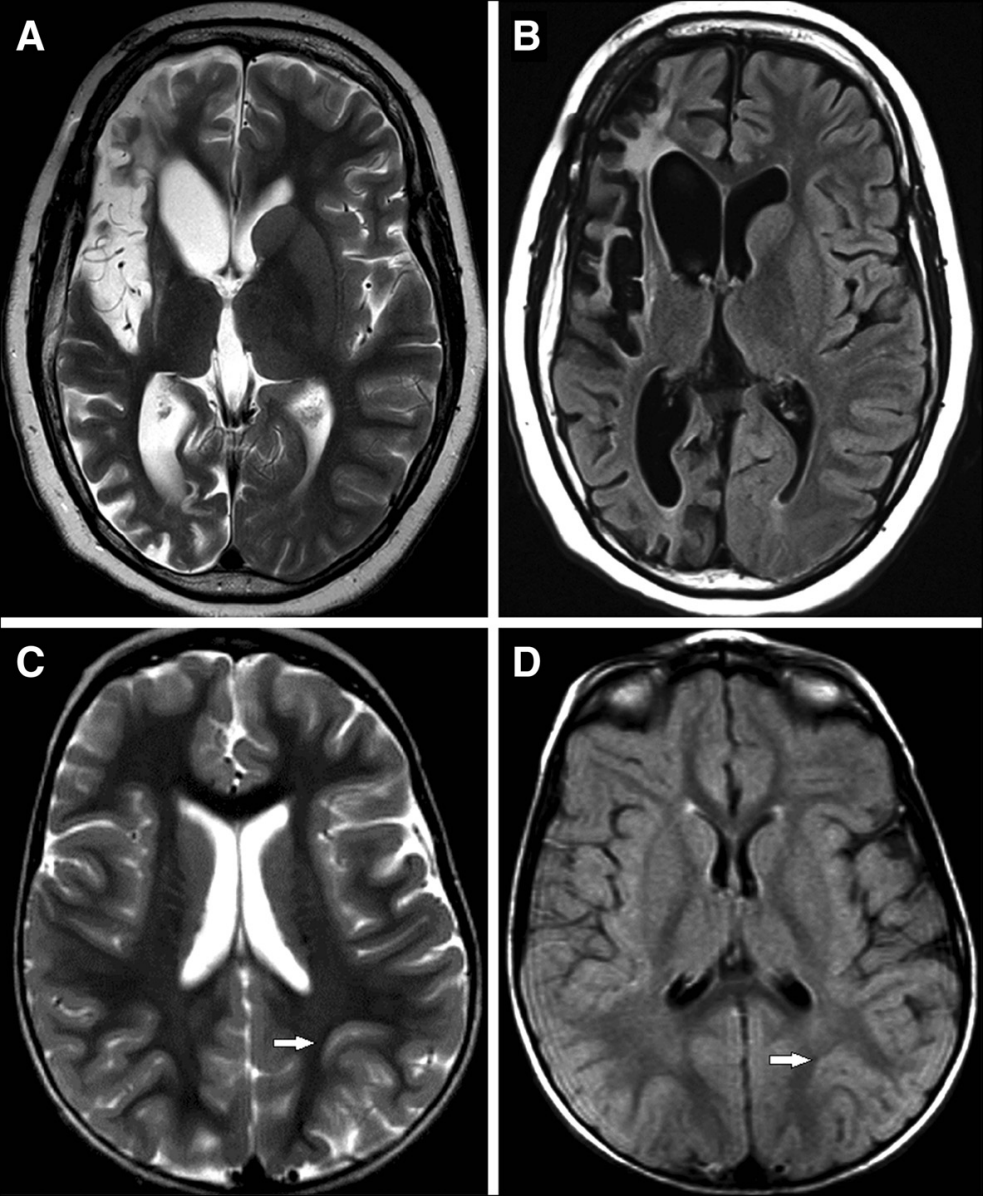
Examples of magnetic resonance imaging (MRI) scans from patients with Rasmussen encephalitis (RE) or cortical dysplasia (CD). Scans were semiquantitatively assessed for T2 (A and C) and FLAIR (B and D) signal changes. The RE case (A and B) was a 13 year old with a five-year history of seizures originating from the right hemisphere. The T2 image was scored severe (4) for tissue destruction (A), and moderate (3) for amount of FLAIR changes (B). The patient with CD (C and D) had abnormality localized to the left parietal area (arrows). The MRI scans were scored zero (no increased signal changes) for T2 (C) and FLAIR changes (D) despite the abnormal anatomy.
